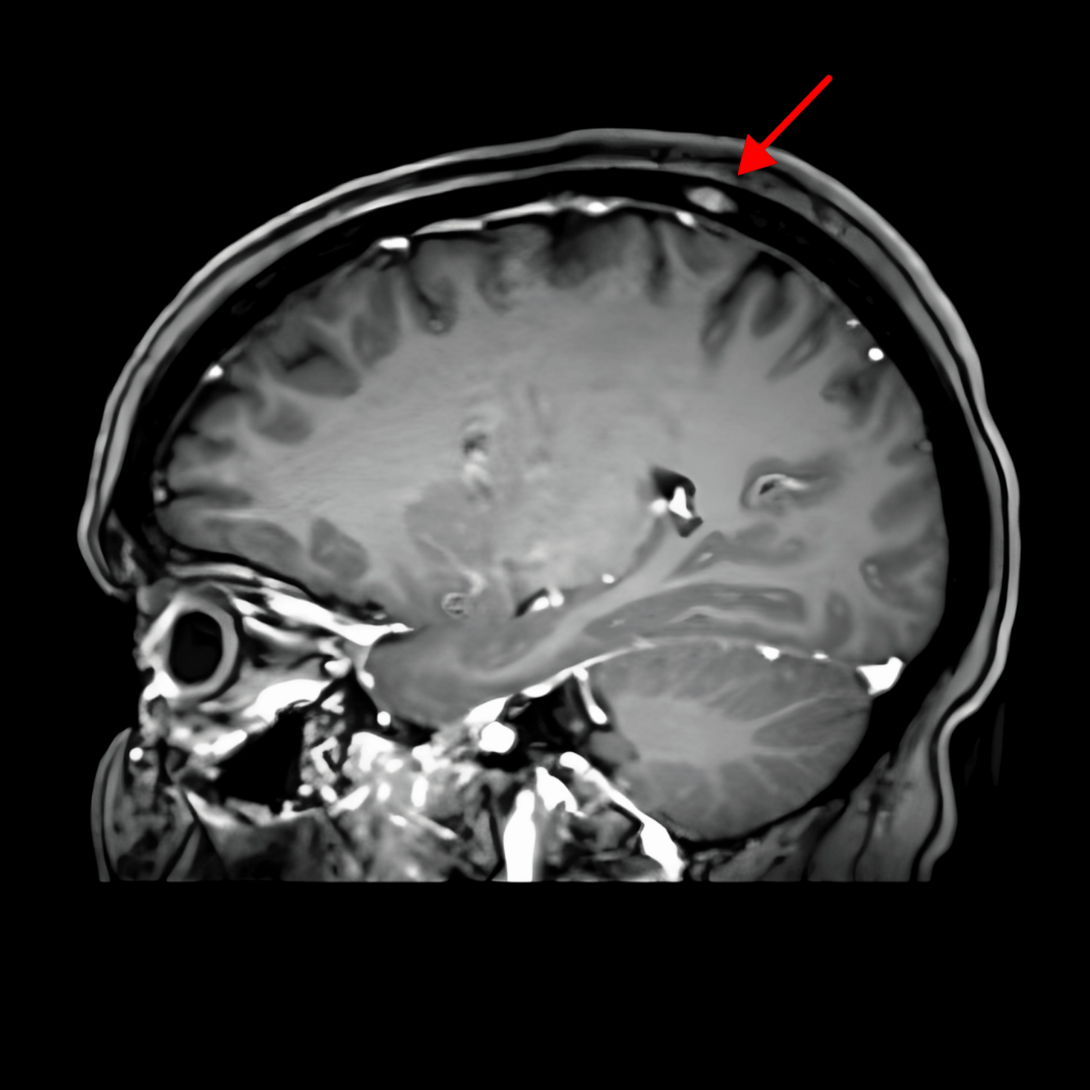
Brain MRI at diagnosis: right parietal bone lesion MRI: magnetic resonance imaging
